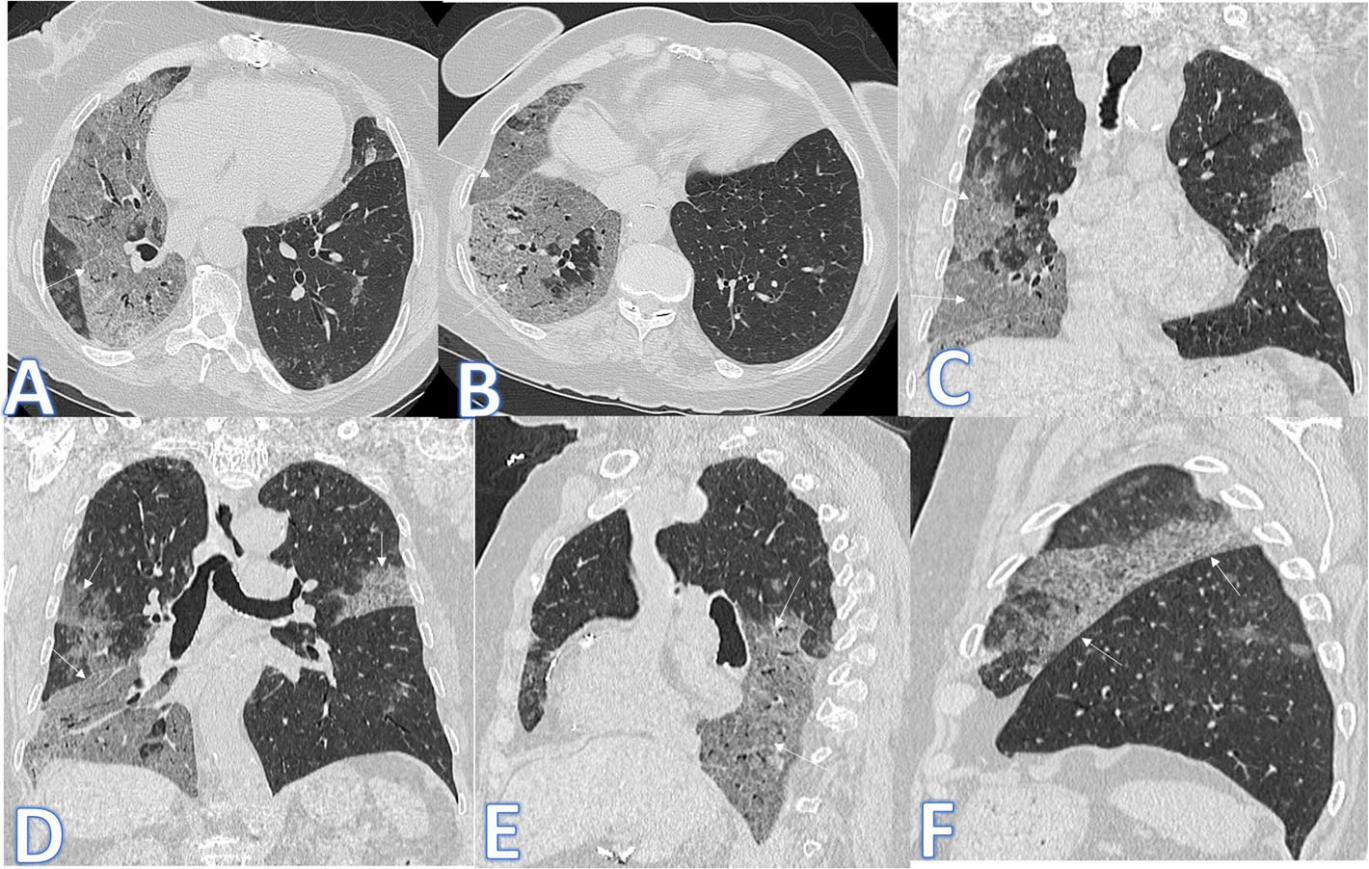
A 80-year-old female patient presented with cough. CT showed bilateral asymmetrical GGO with crazy paving appearance (arrows) near totally involving the right middle and lower lobes with less involvement of the left lung. The diagnosis was PAP
